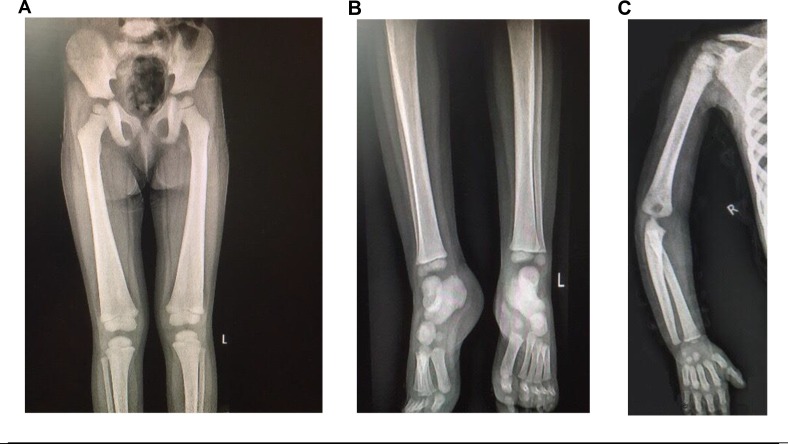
Bone survey. A and B:Radiographs of lower extermeties show femoral metadiaphyeseal widening in Erlenmeyer deformity pattern with endosteal thickenning of femurs and tibias.C:Diaphyseal widening of humerus and ulnrwith symetric increased cortical density.
